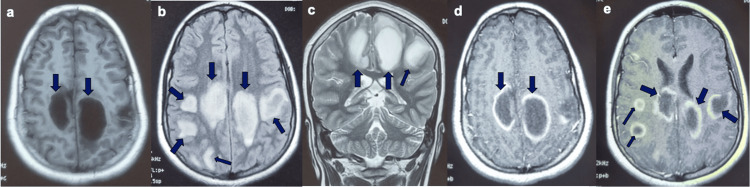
Brain MRI: (a) T1 axial, (b) FLAIR axial (c) T2 coronal, (d) and (e) T1 axial with gadolinium injection
